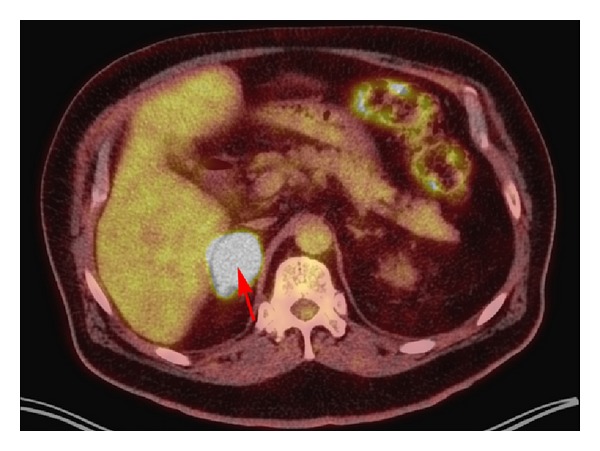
18F-fluorodeoxyglucose positron emission tomography (FDG-PET) scan revealing an intensely metabolic (SUV_max⁡_ = 19.8) right adrenal mass (red arrow), confirming new adrenal metastasis.
